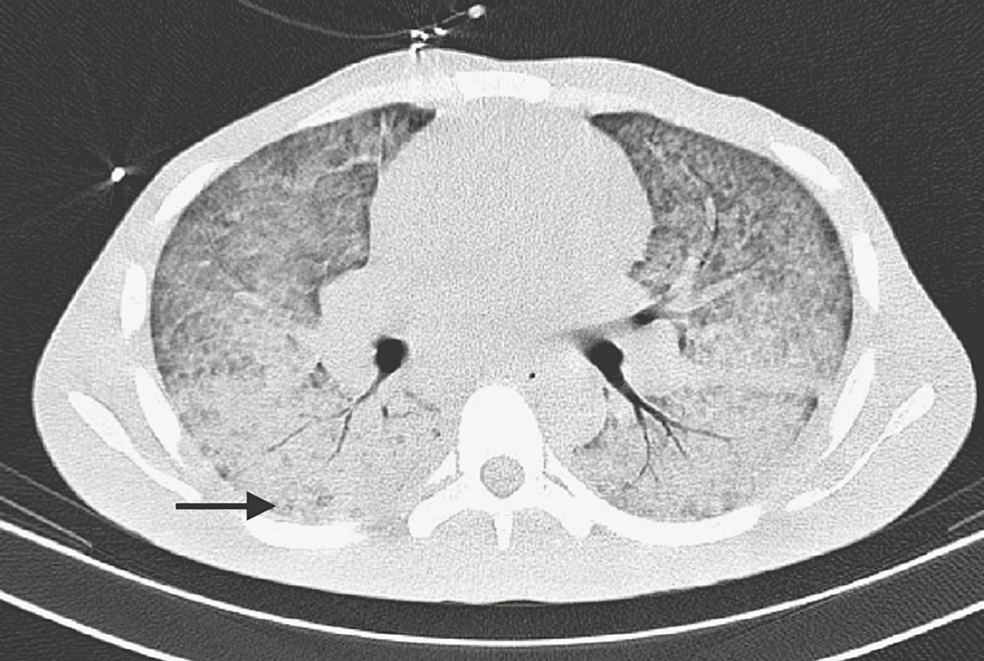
Transversal plane of the thorax computed tomography scan The arrow shows the consolidation of both lungs on ICU admission.
